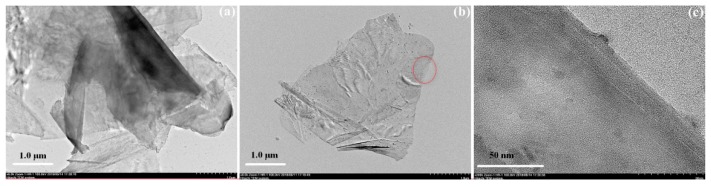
TEM images of graphene after different ultrasonic treatment times: (a) 5 min; (b) 60 min; (c) magnification of red circle marked area of (b).
